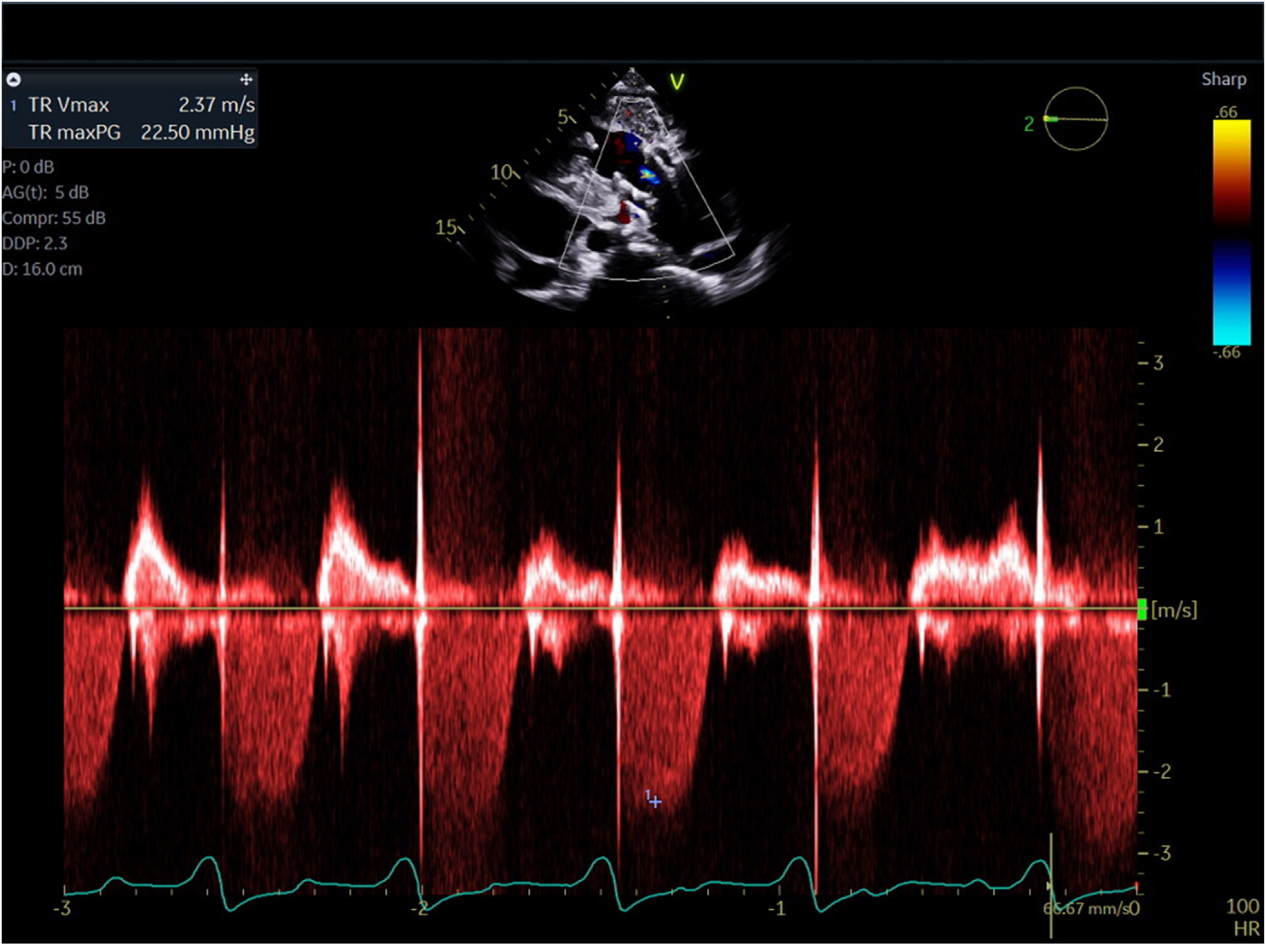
Post INTREPID valve placement transthoracic echocardiogram with tricuspid regurgitation resolution.
